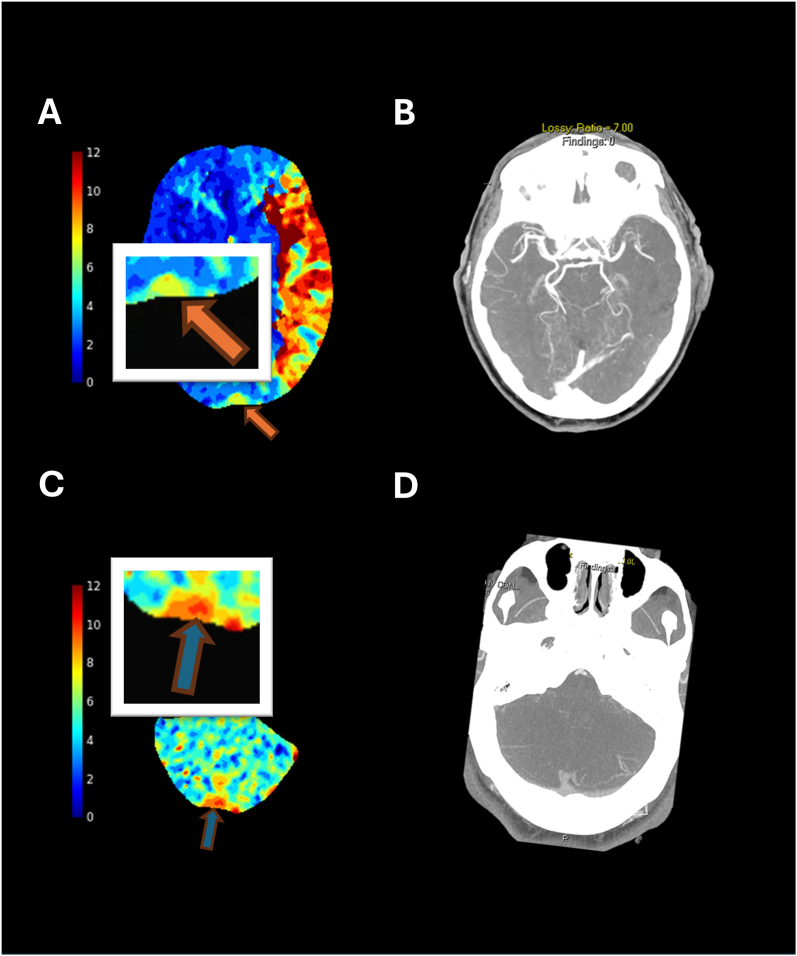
Association between venous outflow status and discharge higher cortical dysfunction (Cog-4) in two patients with anterior circulation large vessel occlusion. (A, B) Images in a 75-year-old man who presented with right facial droop and extremity weakness (admission NIHSS score, 14). (A) Baseline CT perfusion Tmax map shows normal venous transit within the torcula and superior sagittal sinus (orange arrow). (B) CT angiogram source image shows corresponding anatomy. After mechanical thrombectomy (mTICI grade 2b achieved after one pass), the patient demonstrated excellent cognitive recovery at discharge (Cog-4 score, 0). (C, D) Images in a 66-year-old woman who presented with left middle cerebral artery syndrome and high stroke severity (admission NIHSS score, 17). (C) Baseline CT perfusion Tmax map reveals prolonged venous transit, characterized by marked delay in the torcula (blue arrow). (D) CT angiogram source image shows corresponding anatomy. Despite undergoing successful mechanical thrombectomy (mTICI grade 2b after one pass), the patient exhibited significant cognitive impairment at discharge (Cog-4 score, 5).
